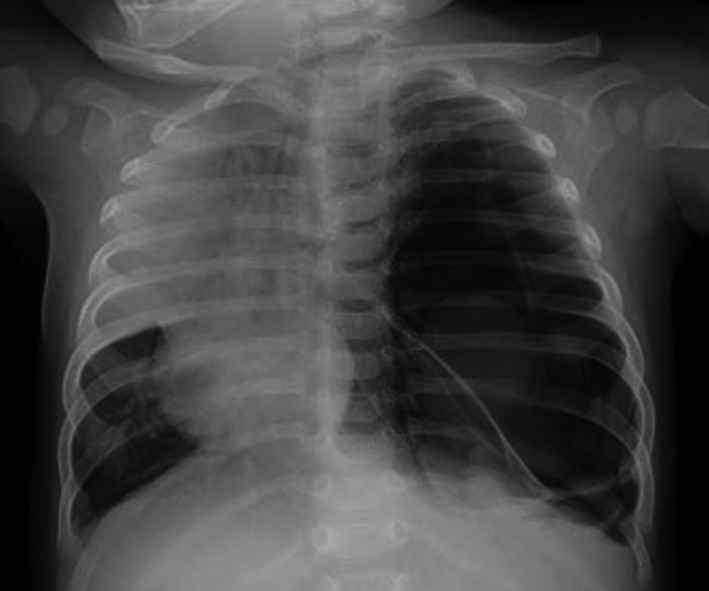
Chest X-ray on the day of transfer. A left thoracic cystic lesion and left pneumothorax are apparent
